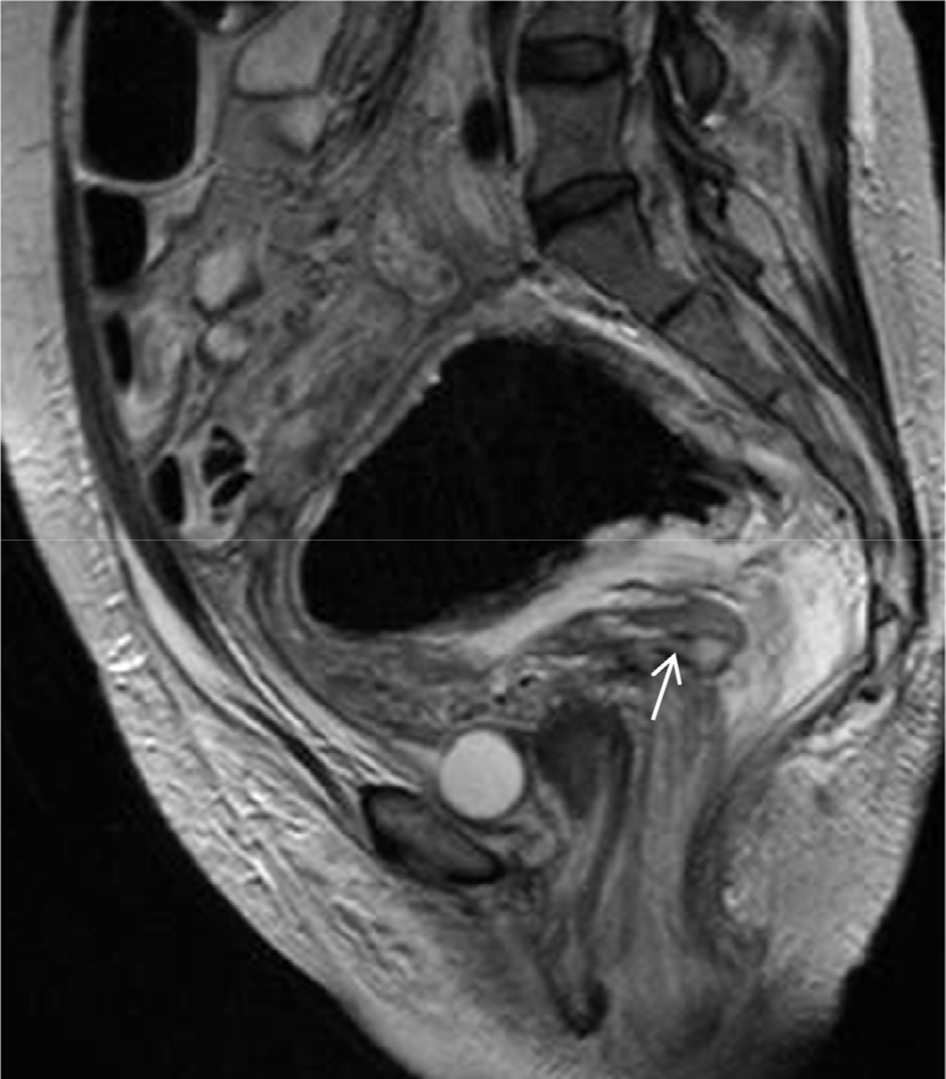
T2-weighted sagittal image: A thick –walled, flask-shaped structure predominately filled with air adjacent to the rectal wall. Arrow shows horizontal low signal intensity line corresponding to the diverticular sinus tract, as confirmed by subsequent CT.
